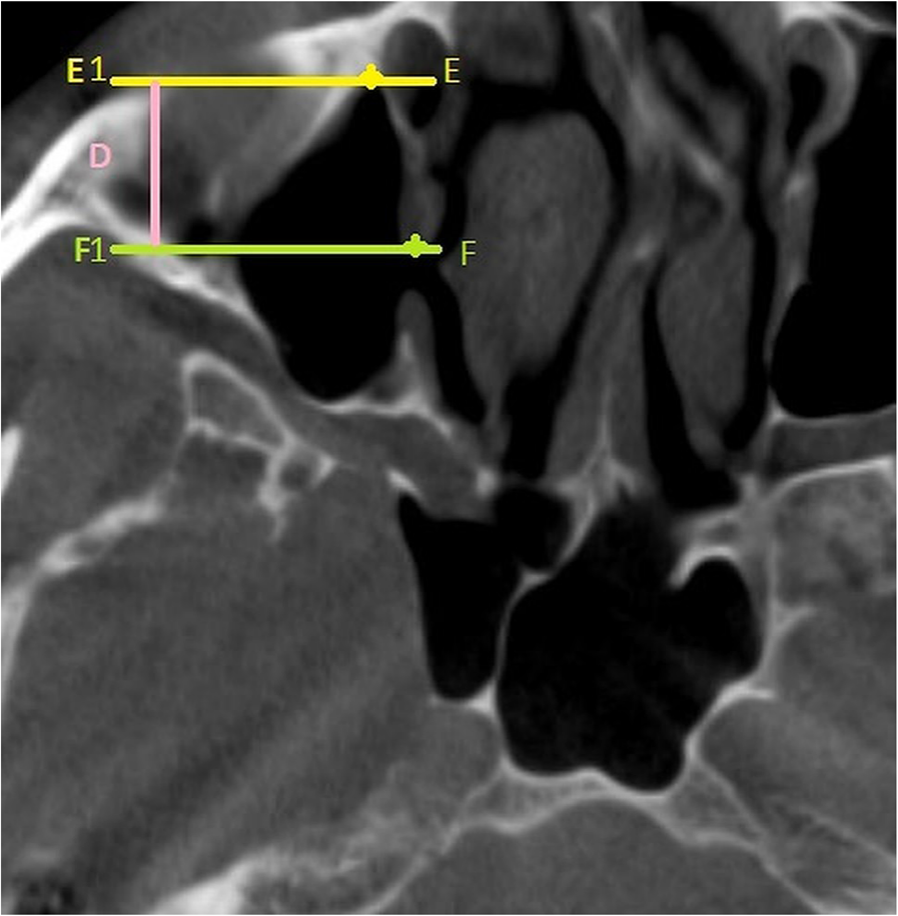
Axial CBCT section showing method used to determine the location of AMO in antero-posterior dimension (D). The distance (D) is obtained by measuring the distance between 2 horizontal lines F1-F (passing through the most anterior point of the AMO) and E1- E (passing through the most anterior point of the sinus)
